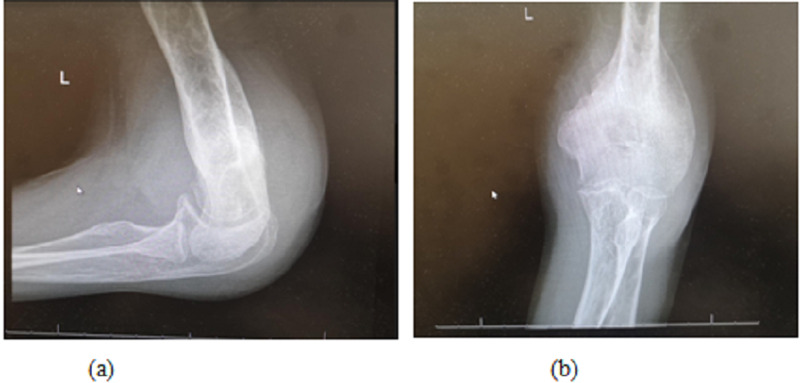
Preoperative Radiographical Images of Elbow lateral view (a) and anteroposterior view (b)
